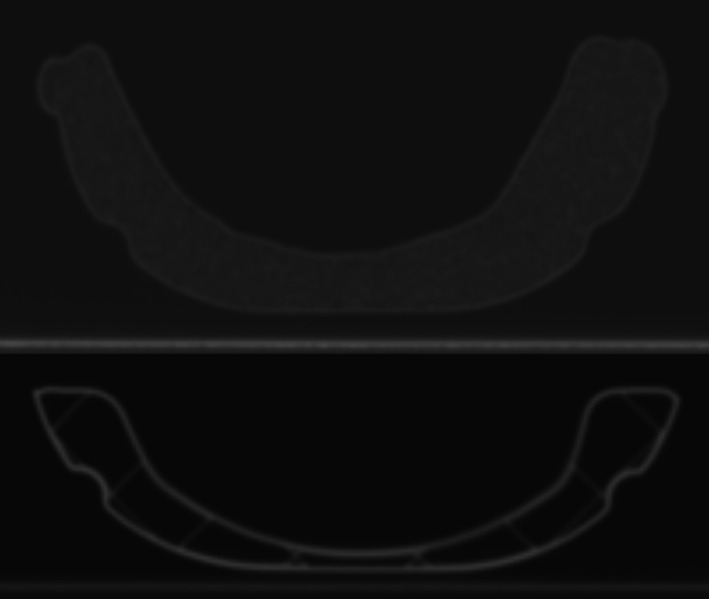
Top: CT scan of standard‐of‐care headrest. Bottom: CT scan of standardized three‐dimensional‐printed headrest.
